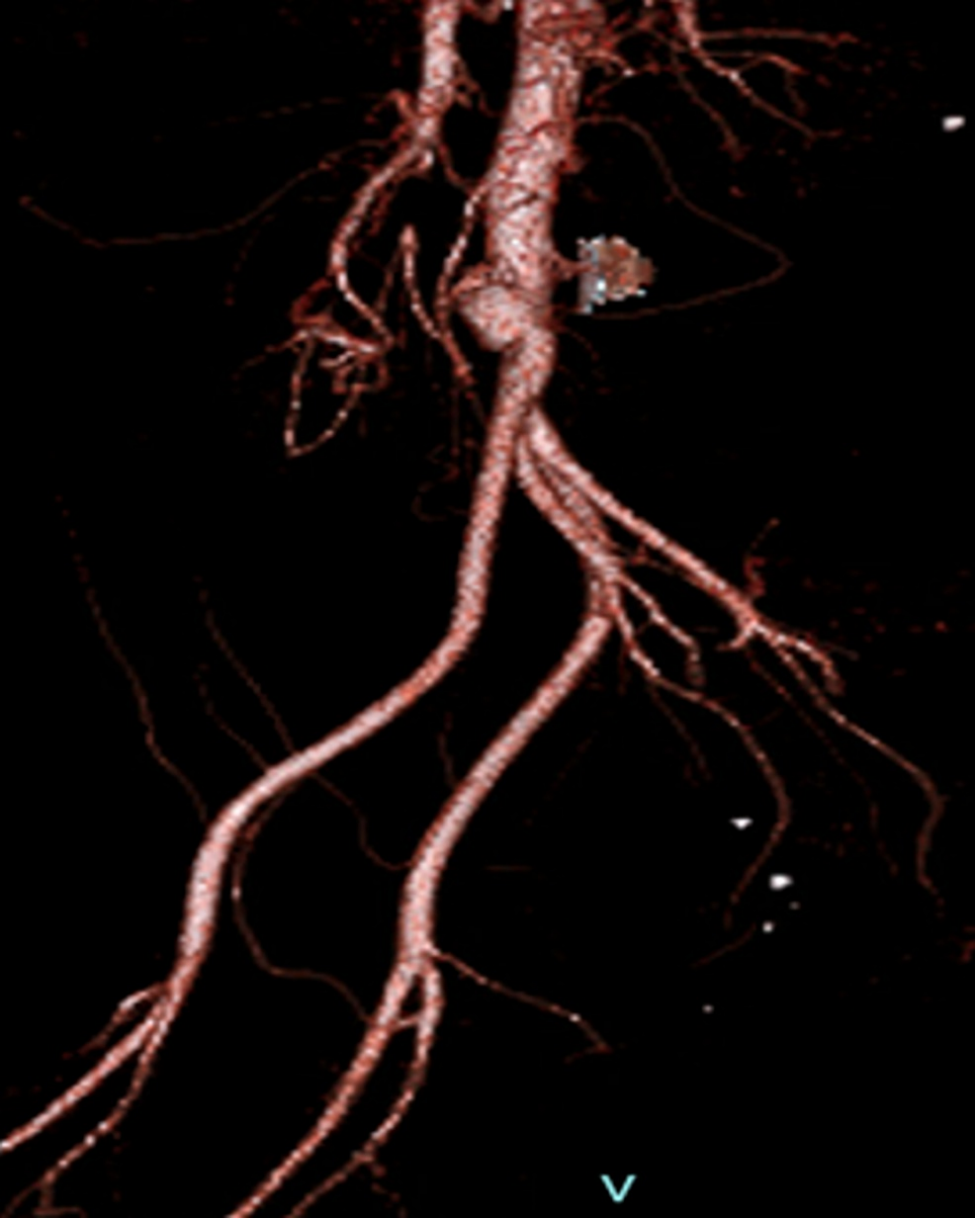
A 3-D reconstituted imaging from CT scan with contrast showing mycotic infra-renal aneurysm
